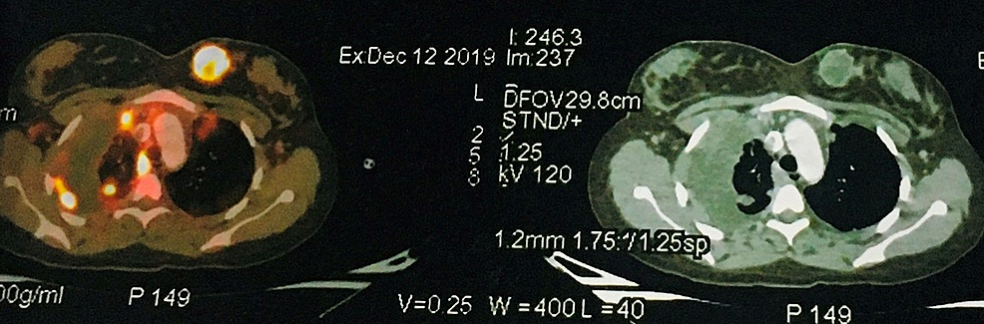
PET-CT scan showing increased fluorodeoxyglucose (FDG) uptake in the inner quadrant of the left breast of size 3.3 x 3.5 cm with SUVmax = 20.9 SUVmax: maximum standardized uptake value
